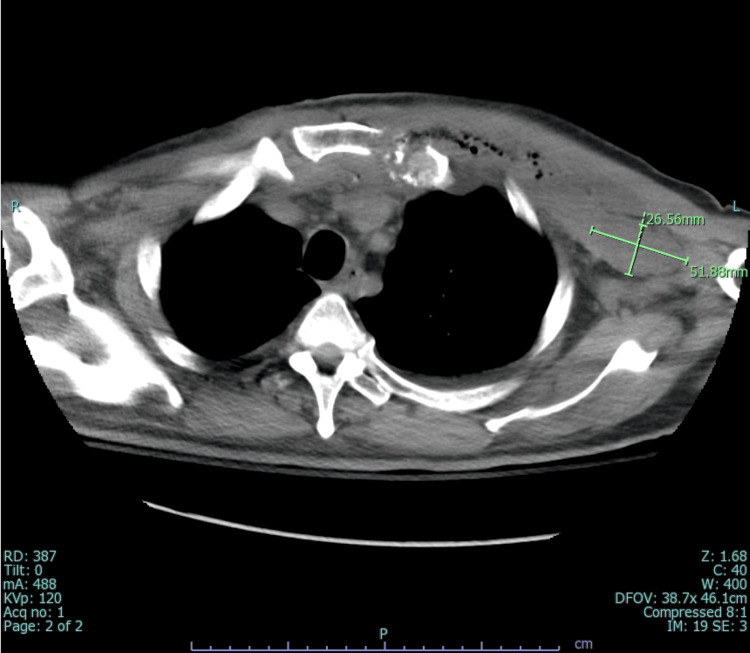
Computed tomography scan (axial view) showing a residual abscess in the left axilla after the first incision and drainage.
